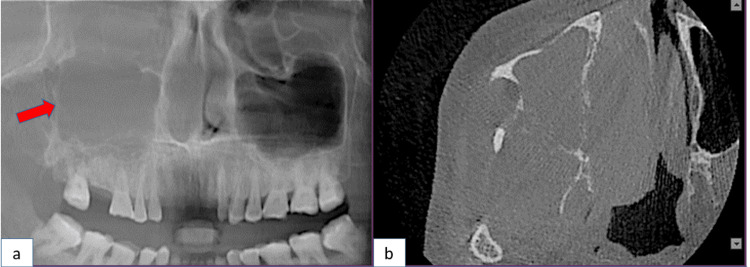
(a) An orthopantomography showing a ground glass appearance, radiopaque in nature. (b) The axial view reveals right maxillary sinus obliteration, along with breach in the posteromedial wall, anterolateral wall, and medial walls of the maxillary sinus.
